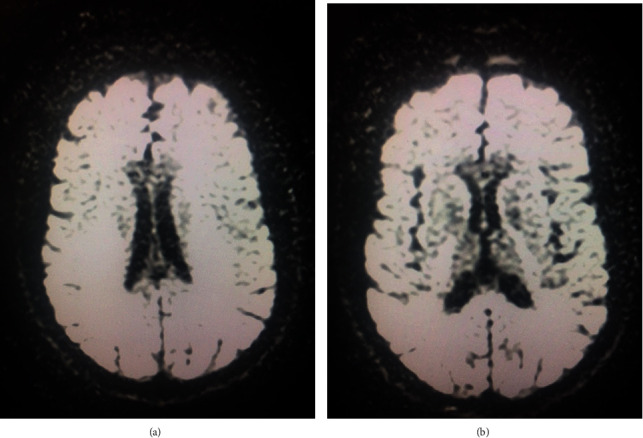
(a) Brain MRI without contrast with diffusion-weighted imaging (DWI). (b) Brain MRI without contrast with apparent diffusion coefficient (ADC).
